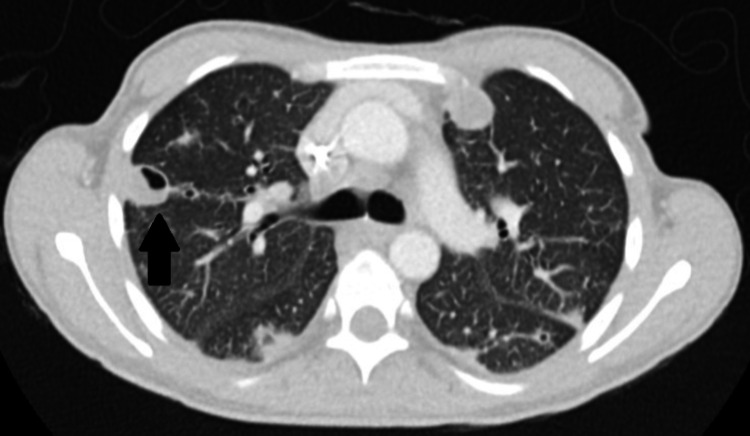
Chest CT with contrast. Chest CT scan demonstrating septic emboli (arrow).
